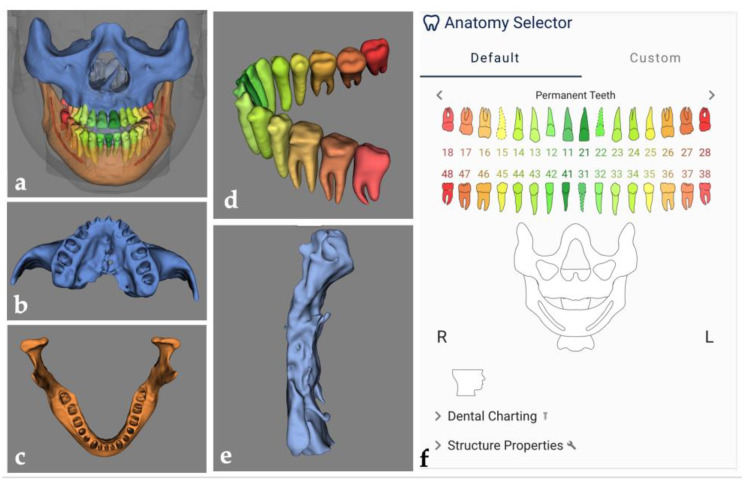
Example of automated segmentation of dento-maxillofacial anatomical structures on CBCT images using a commercially available AI software platform, Relu (Leuven, Belgium; available at https://relu.eu (accessed on 5 December 2022)). The overview of the segmented anatomical structures (a), including the maxilla (b), mandible (c), teeth with orthodontic brackets (d), and pharyngeal airway (e), and automated labeling of teeth (f).
